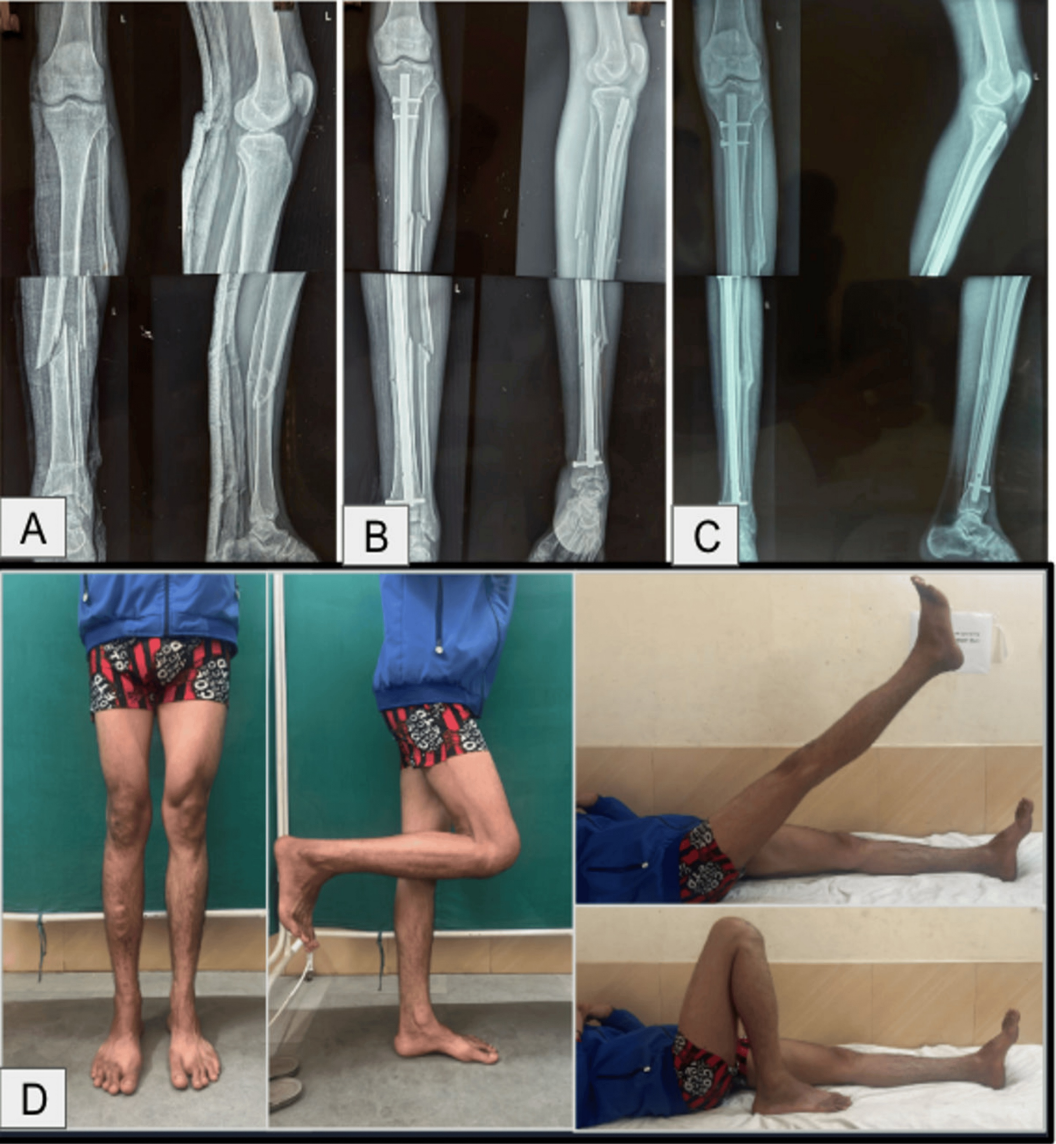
Case 1 of infrapatellar nailing. (A) Preoperative X-ray. (B) Immediate postoperative X-rays. (C) Three months postoperative X-rays. (D) Clinical assessment and knee range of motion at three months.
